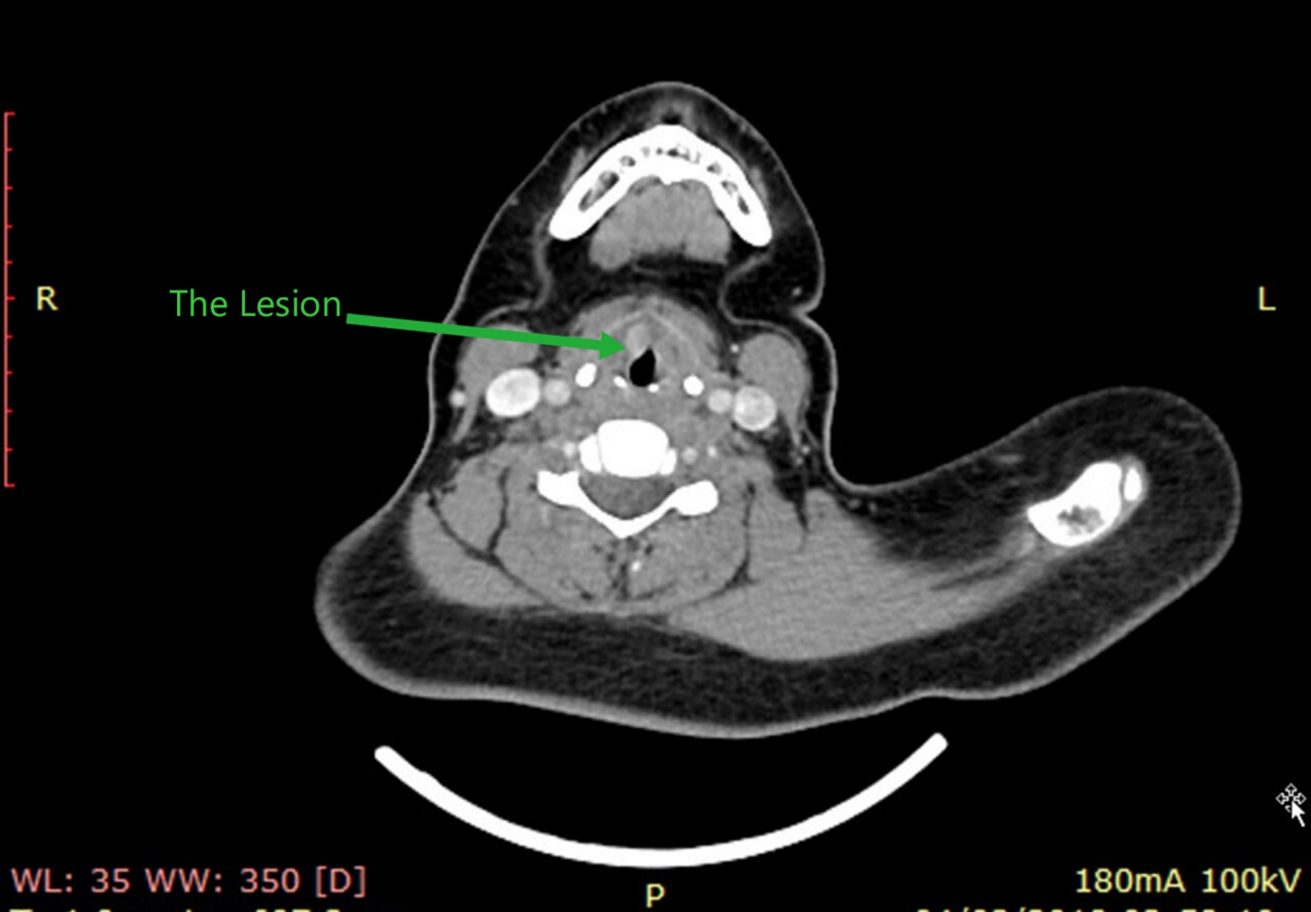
CT scan findings: A lesion at the glottic level, without any lymphadenopathy. CT: computed tomography
